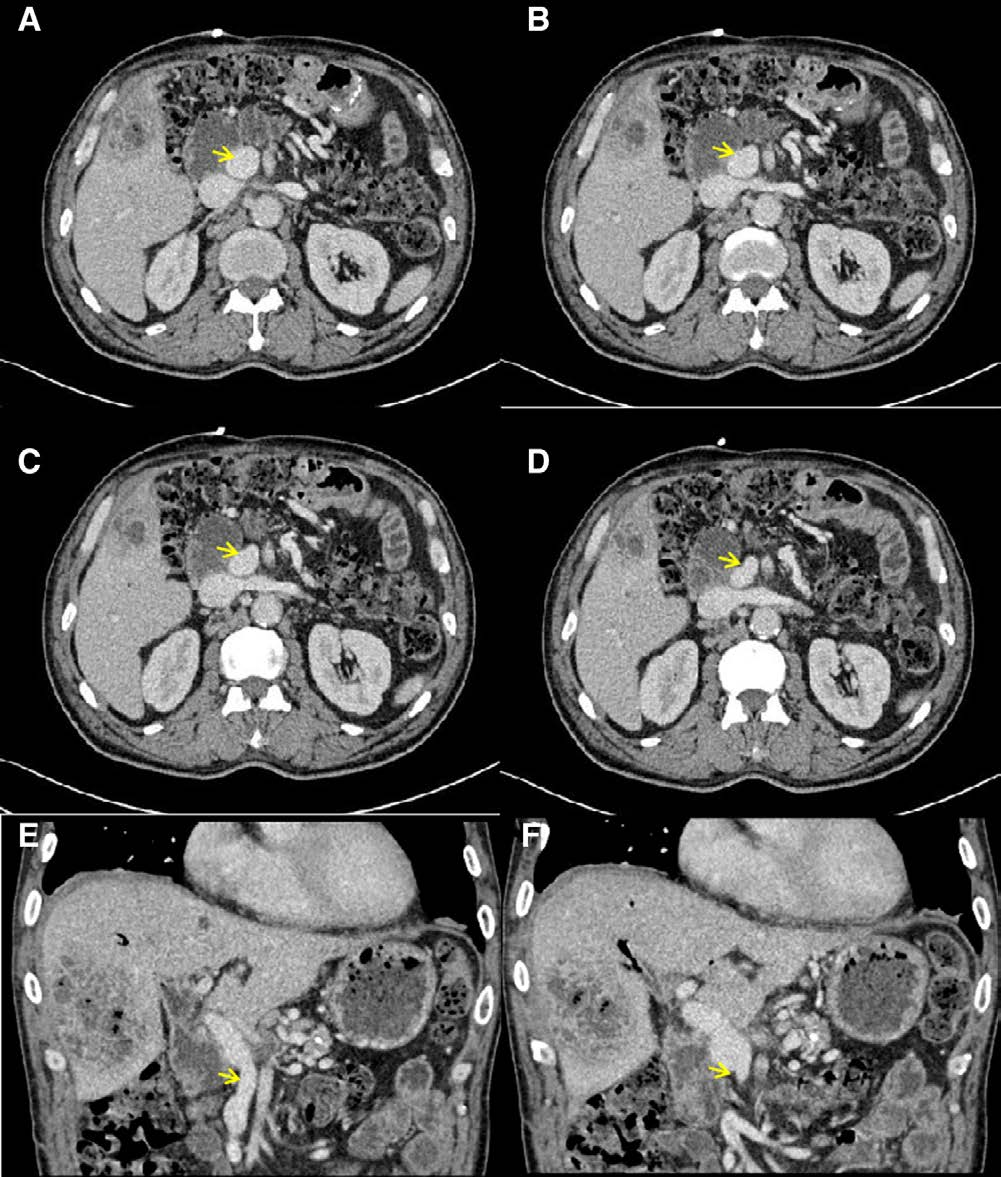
Enhanced CT performed on May 30, 2017. (A–D) Axial enhanced CT image, with the yellow arrow indicating the portal superior mesenteric vein. (E, F) Coronal enhanced CT image, with the yellow arrow indicating the ligamentum teres hepatis graft. CT = computed tomography.
